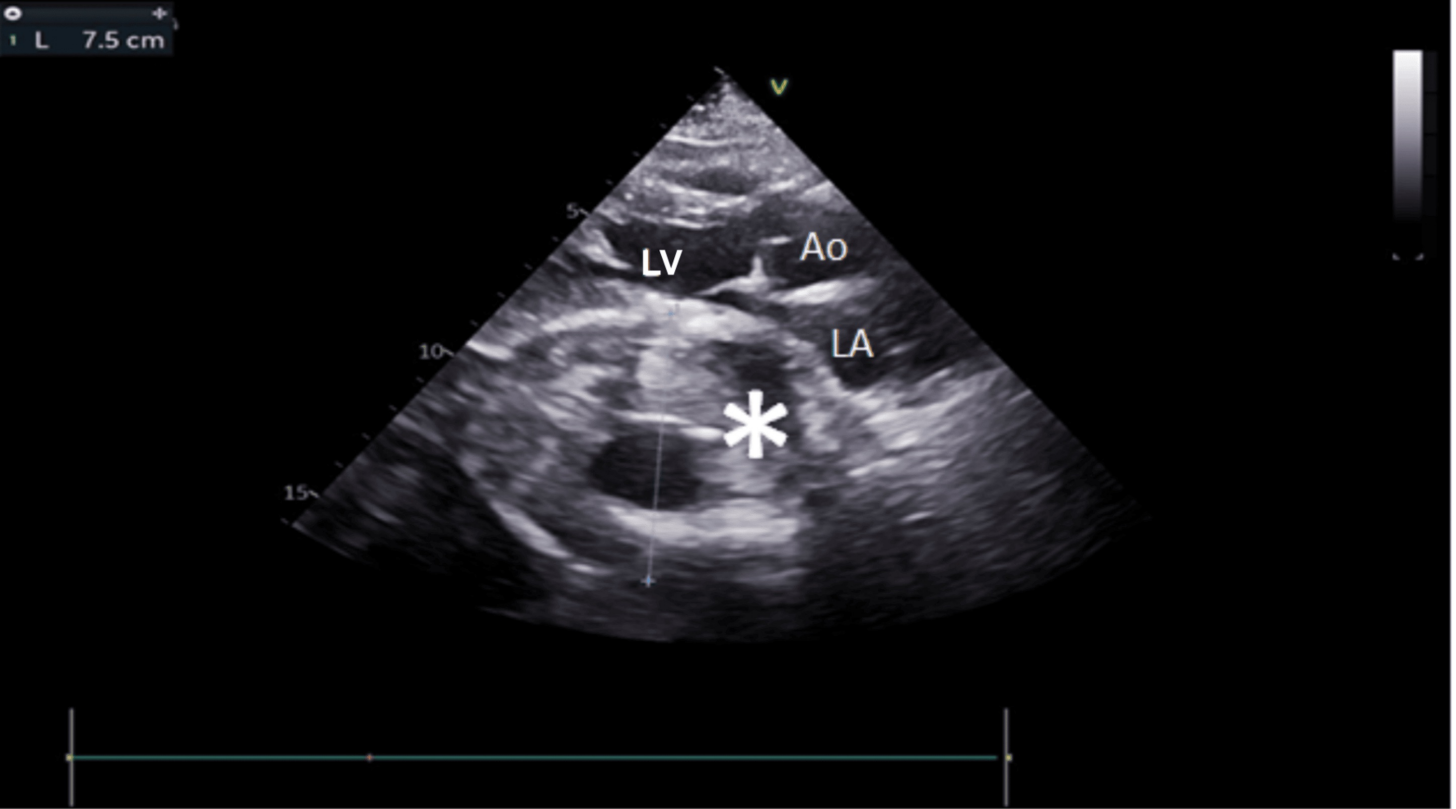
Parasternal long-axis view showing extrinsic compression of the left atrium by a type B aortic aneurysm IMH (asterisk). A left ventricle inflow obstruction is observed. LA: left atrium; LV: left ventricle; Ao: ascending aorta
